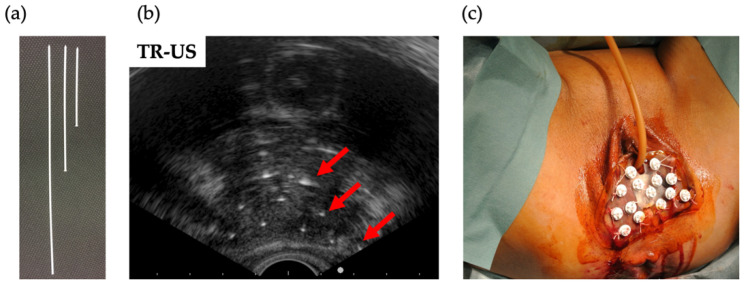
Transrectal ultrasound-guided perineum interstitial needle insertion. (a) One-end plastic interstitial catheter. (b) Red arrows indicate plastic catheters placed 1.5–2.0 cm apart. (c) the perineum after insertion.
